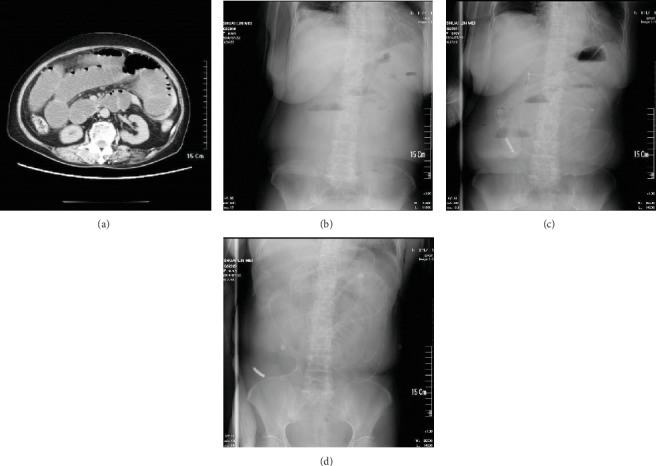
Presentative abdominal X-ray plain films of DIT procedure. (a, b) Before intubation, there were lots of gas-liquid levels, and the proximal intestinal canal dilated obviously. (c) The patient was treated with the DIT procedure, and the intubation depth was up to 265 cm. (d) 24 hours after intubation, the gas-liquid levels had almost disappeared.
